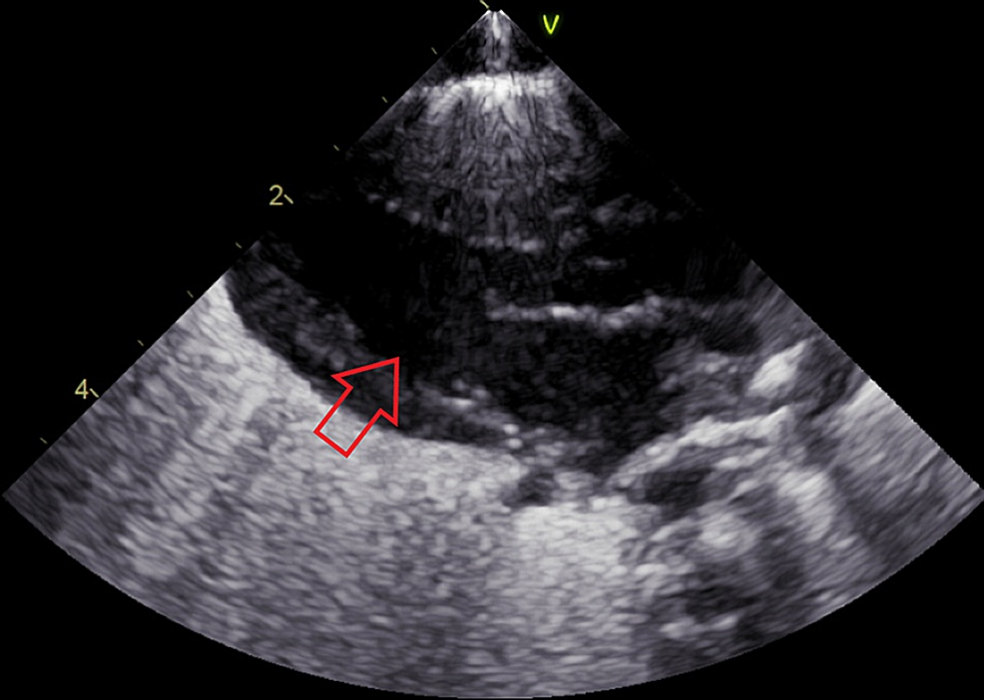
Echocardiogram on day 6 of life shows large patent foramen ovale (arrow)
